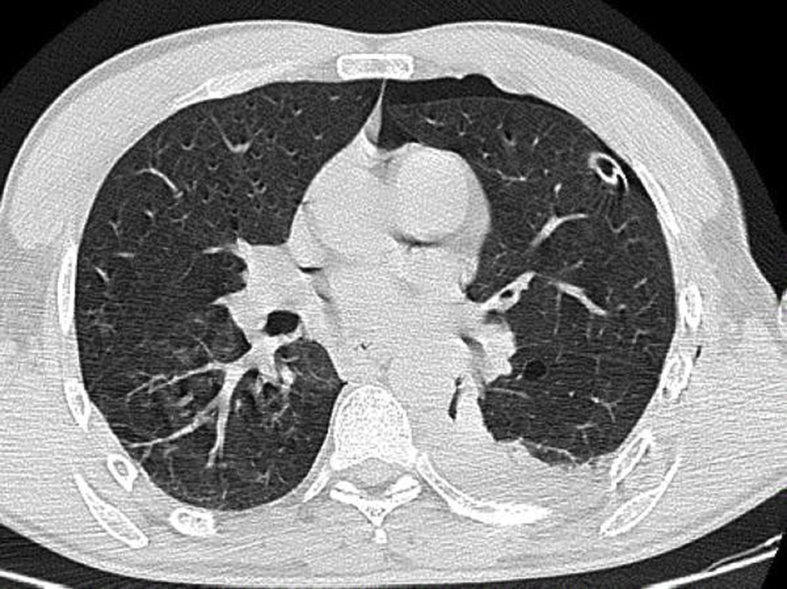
Chest CT scan showing the right-sided 12-F drain in situ and a re-expanded right lung.
